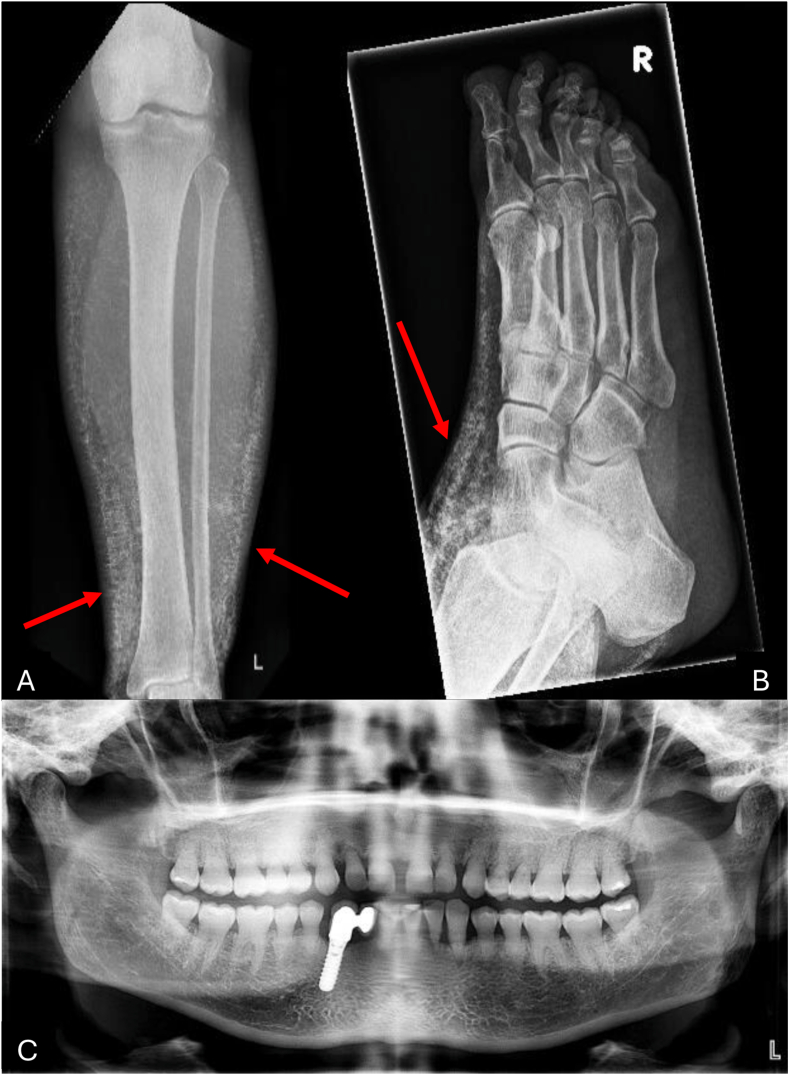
(A) X-ray of the left calf and (B) the right foot showing diffuse cutaneous and subcutaneous calcifications (red arrows). (C) Panoramic dental X-ray depicting teeth with short and bulbous roots and pulp chamber and canal obliterations.
